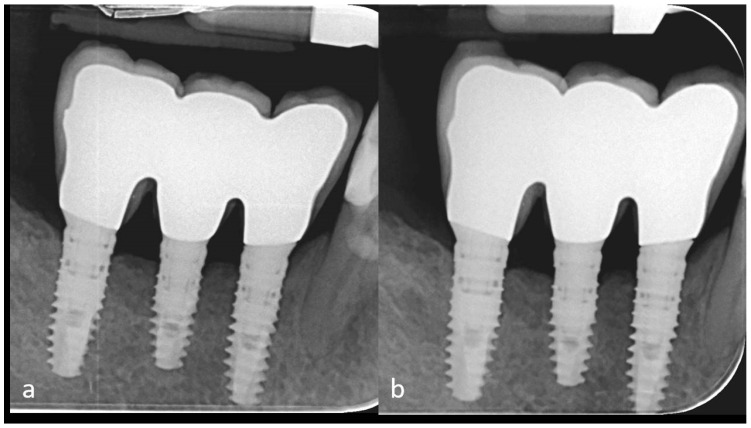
Intraoral radiographies performed 4 years (a) and 6.5 years (b) after implant rehabilitation show good maintenance of the peri-implant bone level.
